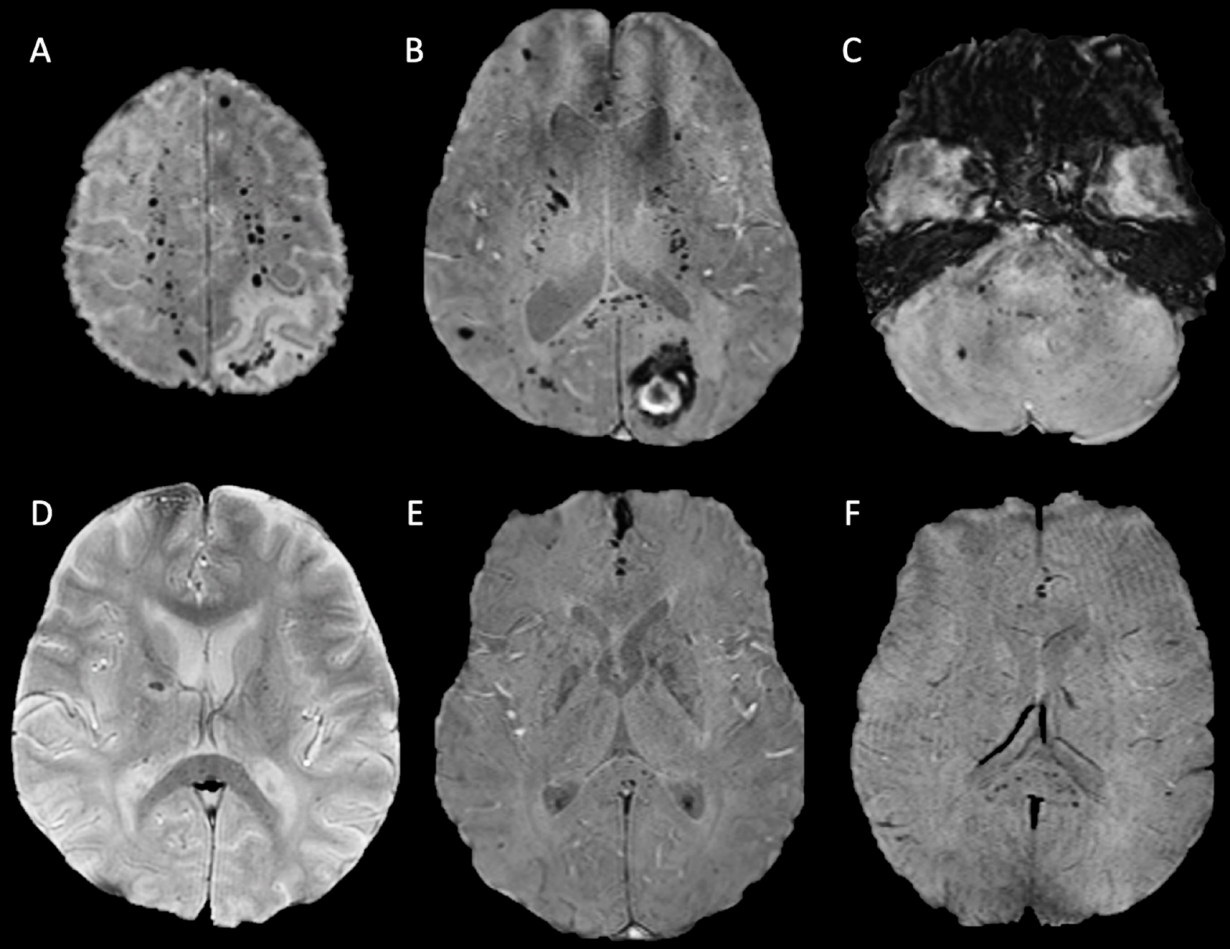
Axial susceptibility-weighted MRI sequences of patient 4 (A–C) and patients 2 (D), 5 (E) and 10 (F). All demonstrating microhaemorrhages in the splenium of the corpus callosum and juxtacortical and subcortical white matter. Patients 4 (A–C) and 2 (D) also both exhibit microhaemorrhages in the internal capsule. The axial image of the posterior fossa in patient 4 (C) demonstrates further microhaemorrhages in the pons, middle cerebellar peduncles and cerebellum.
